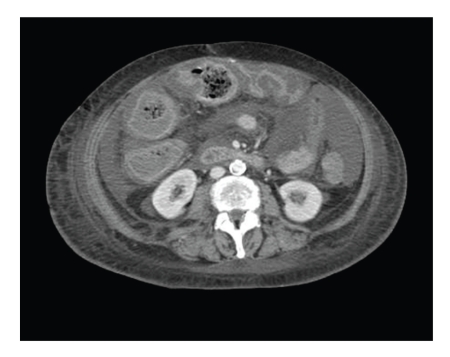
A CT-scan of the abdomen showing increased thickness of the colonic wall.
